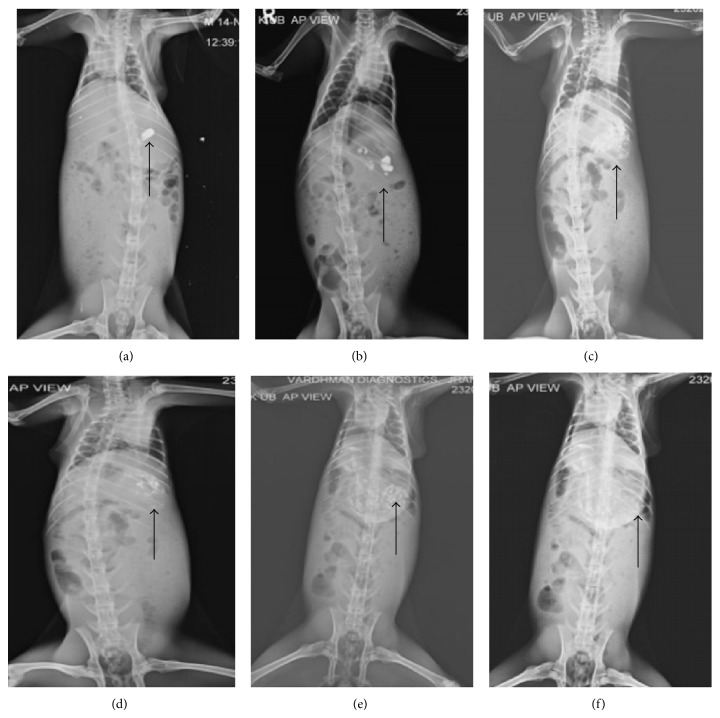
Radiographic images showing the presence of BaSO_4_ loaded floating hollow microspheres in the stomach at different time intervals. Images were taken after (a) 0 hr, (b) 1 hr, (c) 2 hr, (d) 3 hr, (e) 4 hr, and (f) 6 hr.
